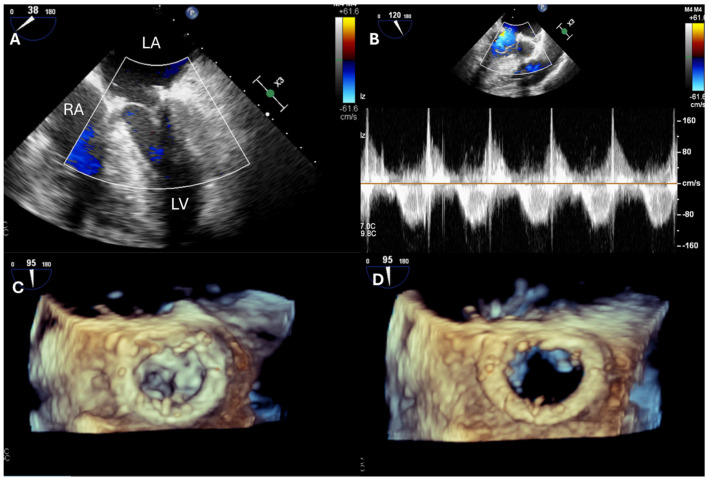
Postoperative assessment of a patient undergoing mitral valve repair with chord implantation and ring placement for primary MR. (A) Using color Doppler, TEE can assess for residual MR or the presence of paravalvular leaks, which were excluded in this case. (B) With continuous Doppler, the transmitral flow is recorded, and the mean gradient can be measured. (C,D) Using a 3D view, TEE can visualize the mitral valve from the “surgical views” with the aorta in the 12th h and the left atrial appendage in the 9th h. In this case, no residual flail segment was noted, and the opening of the mitral valve was also anatomically sufficient. LA: left atrium; LV: left ventricle; MR: mitral regurgitation; RA: right atrium.
